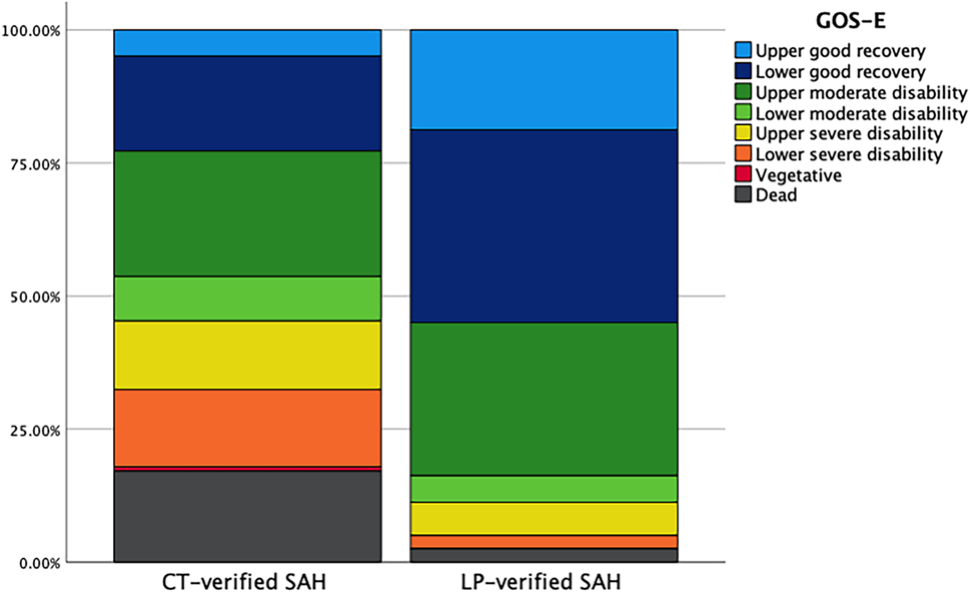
Clinical outcome for the CT- and LP-verified SAH cases 1-year post-ictus. The figure demonstrates clinical outcome (GOS-E) 12 months post-ictus for those with CT- and LP-verified SAH. Those with a CT-verified SAH had a higher rate of mortality (17% vs. 3%), vegetative state (1% vs. 0%), lower severe disability (15% vs. 3%), upper severe disability (13% vs. 6%), and lower moderate disability (8% vs. 5%), whereas they had a lower rate of upper moderate disability (24% vs. 29%), lower good recovery (18% vs. 36%), and upper good recovery (5% vs. 19%). CT = computed tomography. GOS-E = Glasgow Outcome Scale-Extended. LP = lumbar puncture. SAH = subarachnoid hemorrhage
